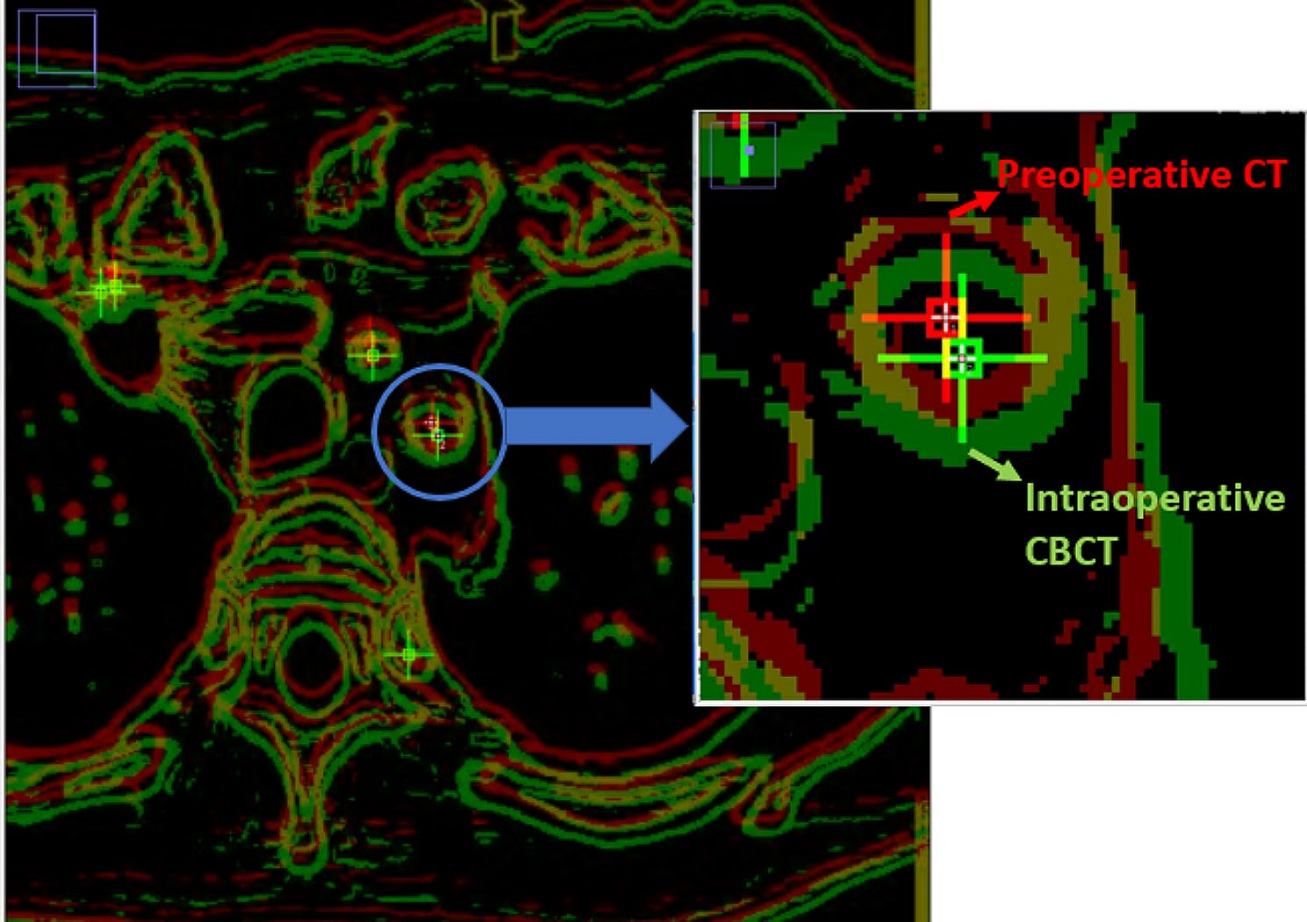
The distance between the center of LSA ostium between preoperative CTA (red cross cursor) and intraoperative CBCT (green cross cursor) imaging was measured using ImageJ. The deviation derived from automatic bony-based registration and soft tissue-based registration method could be computed, which indicated the improvement of mismatch in terms of the ROI (LSA ostium) in using novel soft-tissue method
